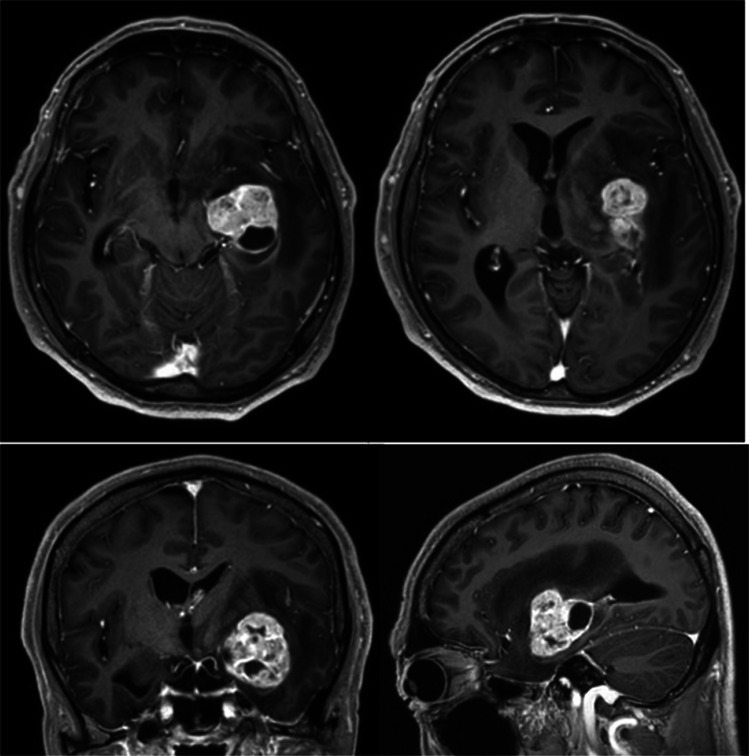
Preoperative axial (upper panel), coronal (lower left panel), and sagittal (lower right) gadolinium-enhanced T1-weighted magnetic resonance images showing a heterogeneously enhancing lesion in the left medial temporal lobe adjacent to the basal ganglia (BG). The exact boundary of the tumor and its invasion into the BG remain unclear
